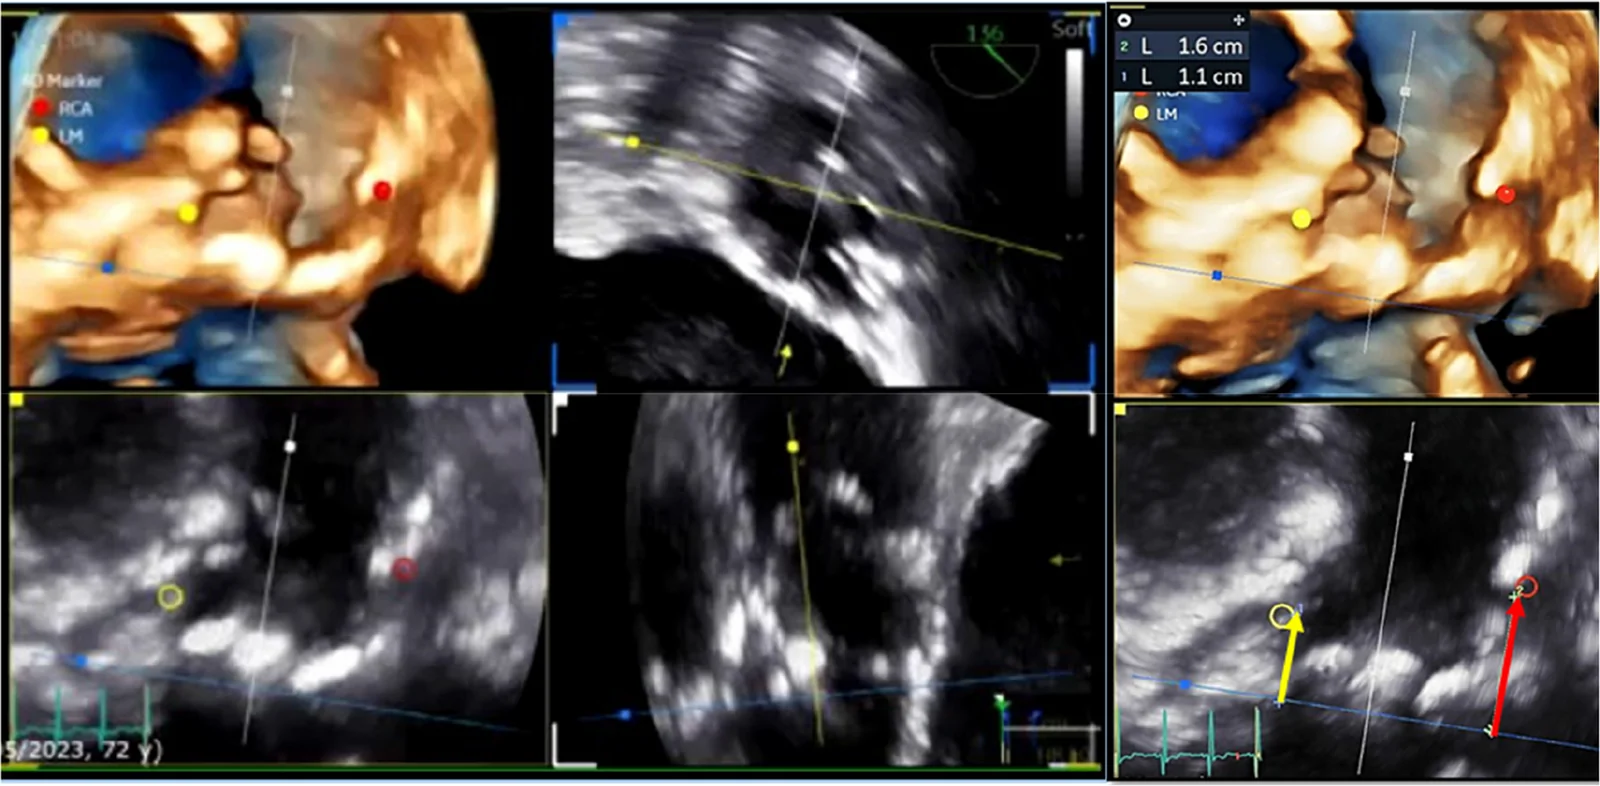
Multiplanar imaging for coronary height measurements with aid of markers on 3D transesophageal echocardiography (TEE). Left and right coronary artery ostia are marked as the yellow and red circles, respectively on 3D TEE dataset. Using 3D multiplanar reconstruction and the markers, the height of both coronary artery ostia are easily identified on the volume rendering view. In this case, the left coronary ostium is measured at 11 mm, while that of the right coronary ostium is 16 mm above the annular plane, respectively
